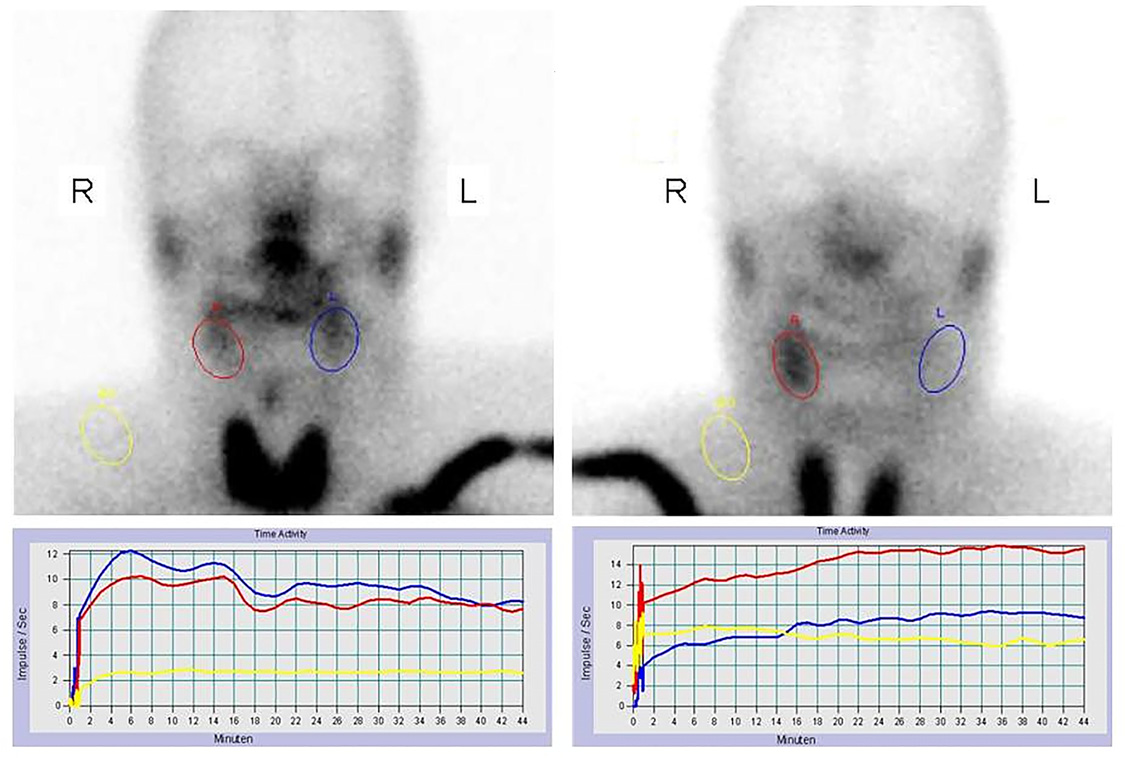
Salivary gland scintigraphy before (left) and after (right) radiotherapy of patient number 11. A consistently higher uptake level of the right submandibular gland area treated with BoNT compared to the control gland on the opposite side was demonstrated. Time activity curves on quantitative scintigraphy of the submandibular gland before (left) and after (right) radiotherapy are shown. Red: right gland, blue: left gland, yellow: background activity.
